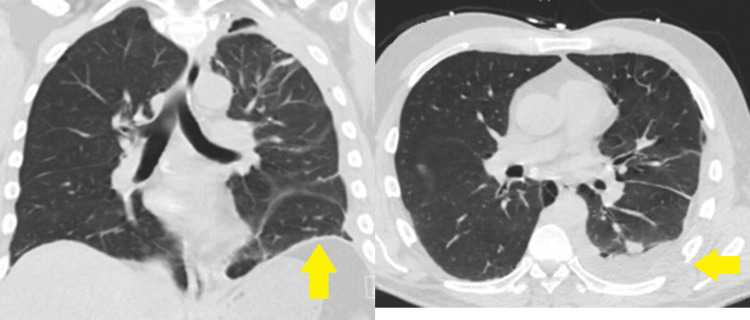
CT scan 10 days post thoracotomy showing near-complete resolution of the left-sided hemothorax and retropleural hematoma along with re-expanded left lung.
